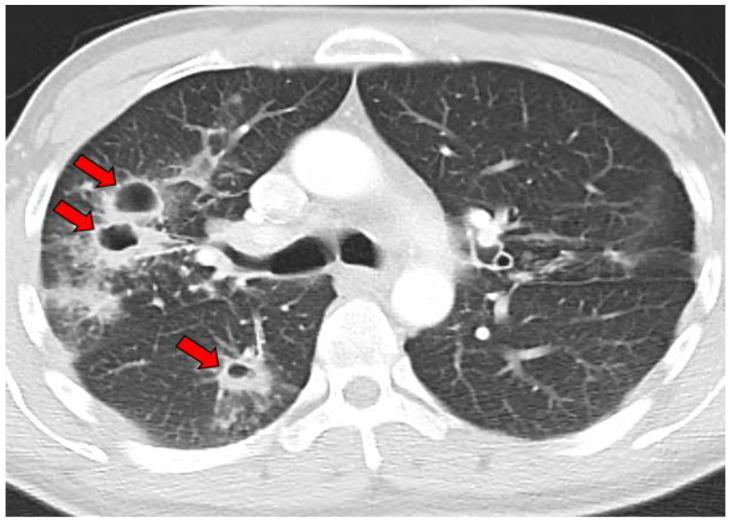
CT image of multiple coccidioidal pulmonary cavities. The red arrows indicate cavities.
